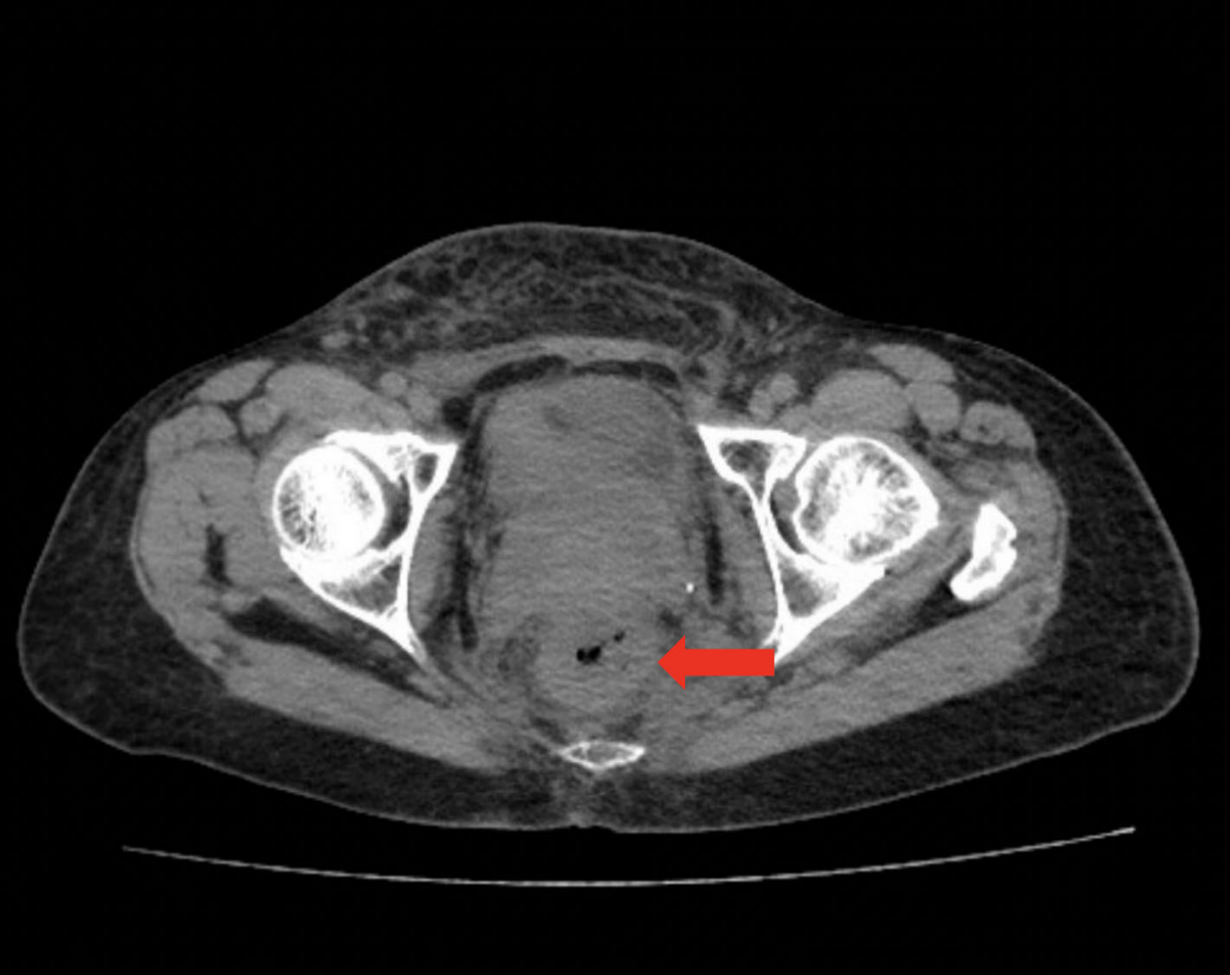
CT imaging of rectal metastasis. Red arrows indicate the site of metastatic involvement in the rectum, characterized by significant thickening of the bowel wall. The examination was performed on August 31, 2024, using an abdominal non-contrast CT scan.
